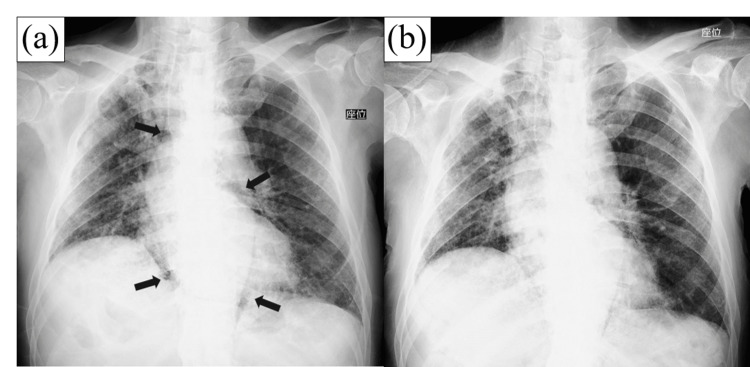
(a) Chest X-ray during mediastinal emphysema exacerbation (arrows). (b) Chest X-ray after mediastinal drain insertion. The drain was placed in the mediastinum, and emphysema subsequently resolved.
